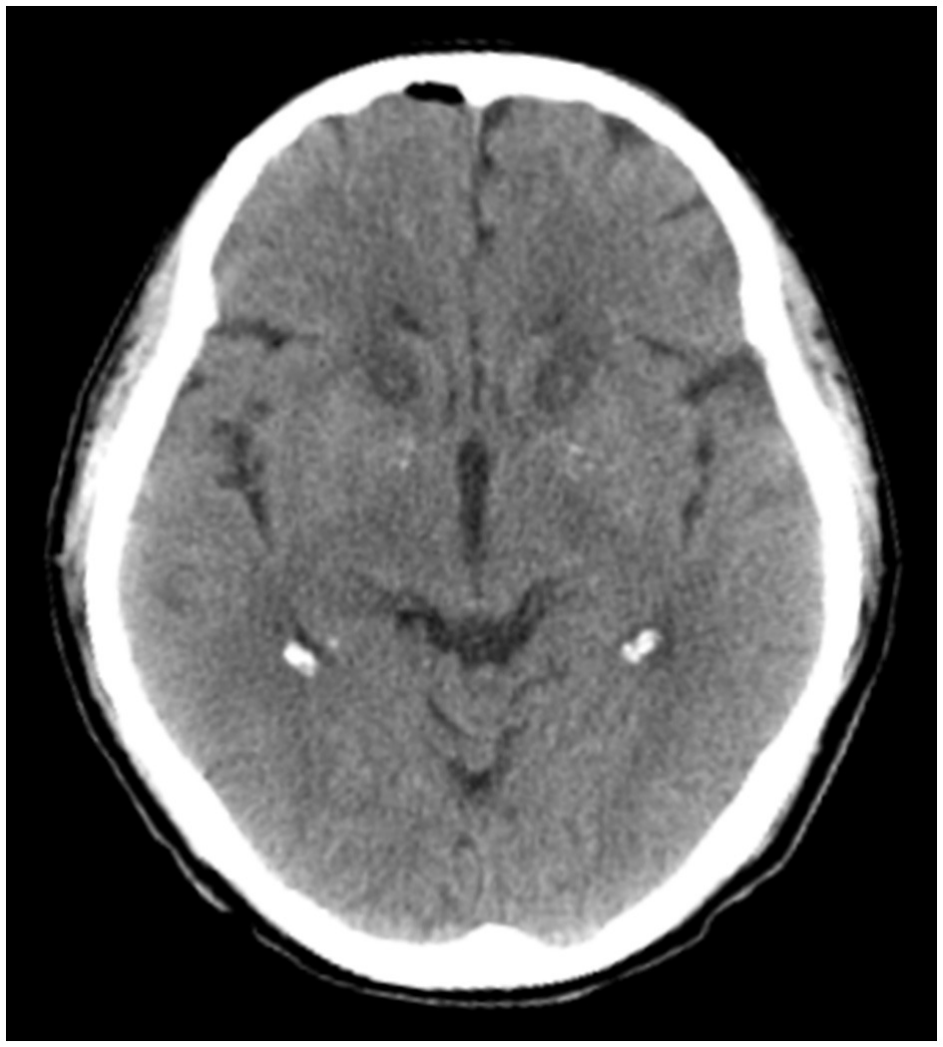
A computed tomography slice of representative ALIC lesions created by bilateral anterior capsulotomy, taken on postoperative day 1.
